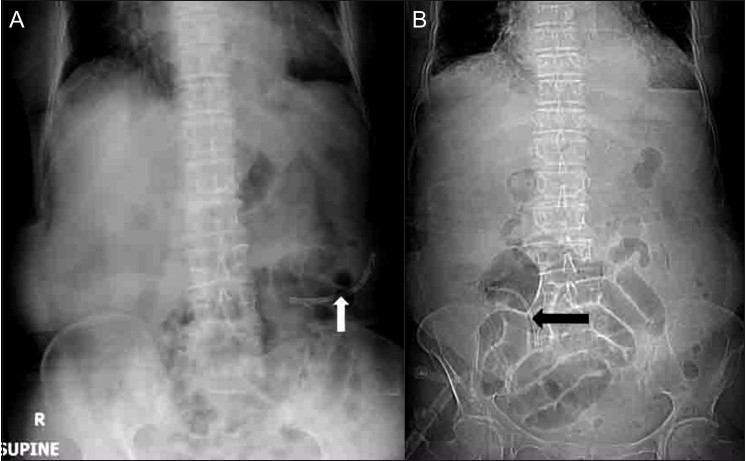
Supine plain abdominal radiographs obtained two days before presentation (A) and on the day of admission (B) shows a migrated stent in the left iliac region (white arrow in A), which then shows further migration into the right iliac fossa (black arrow in B), with dilated small bowel loops in the lower abdomen and pelvis (B)
